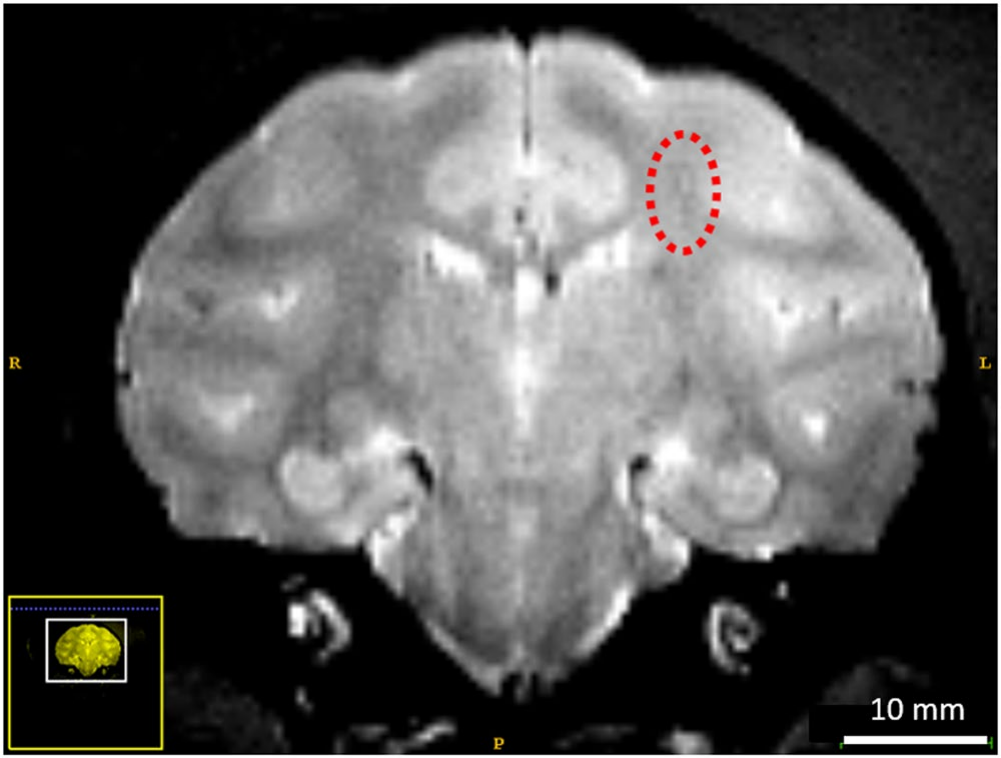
T2-weighted images one month after BBB opening shows a suspicious contrast in the focal region (dashed circle). No residual BBB leakage was detected.
